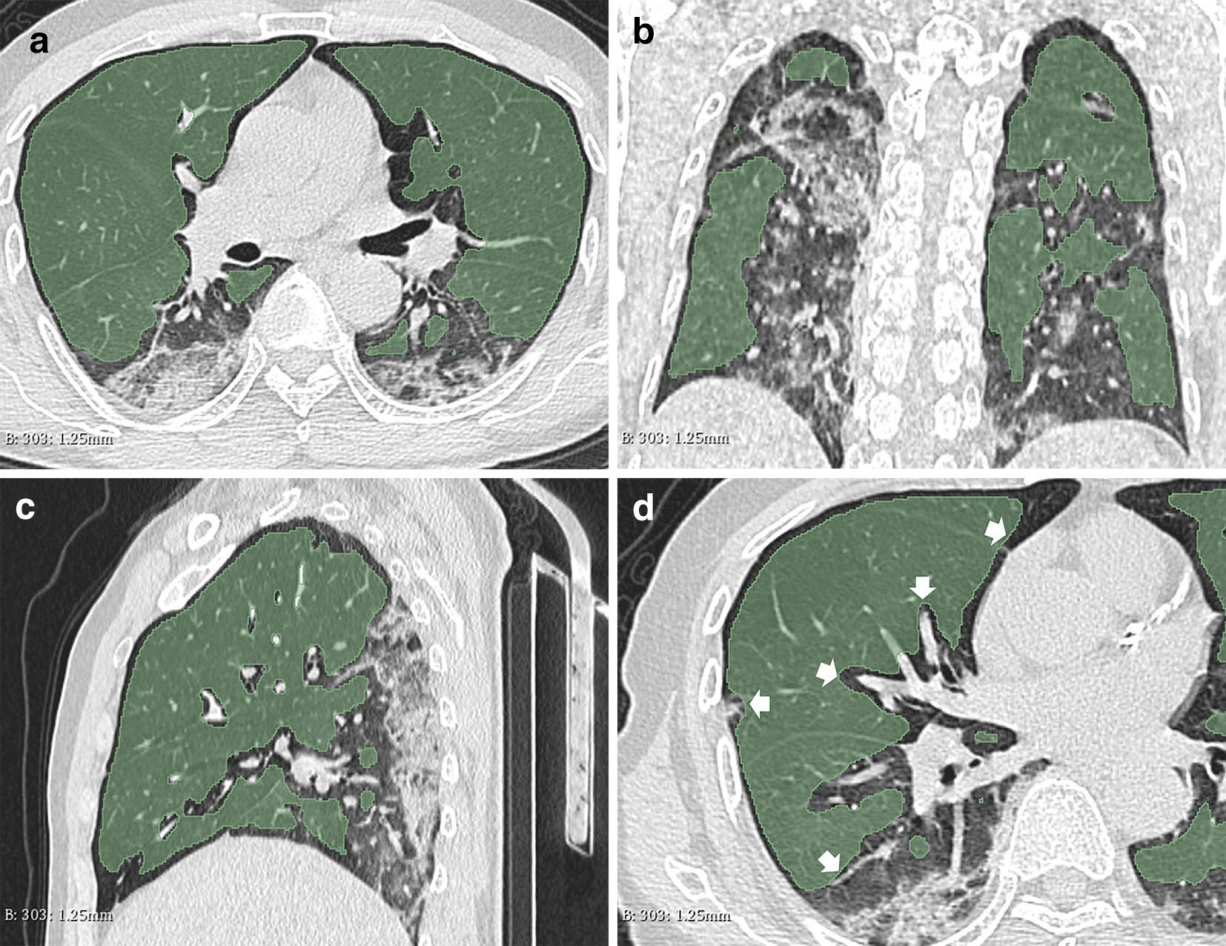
(a–d) Axial (a), coronal (b) and sagittal (c) of CT images, showing the VOI range of segmented images. The region growing method was used to segment VOI on 3D-slicer software. By adjusting the threshold range of CT value to exclude the pulmonary vessels and bronchi above the second grade in the lung, Gauss smoothing was used to reduce the edge of VOI by 3 mm, avoid overlapping with the focus and disturb the analysis results. Typical image layers of axial, coronal and sagittal plane of the same patient's CT image (a–c); “White arrow” marks the boundary of artificial shrinkage (d).
